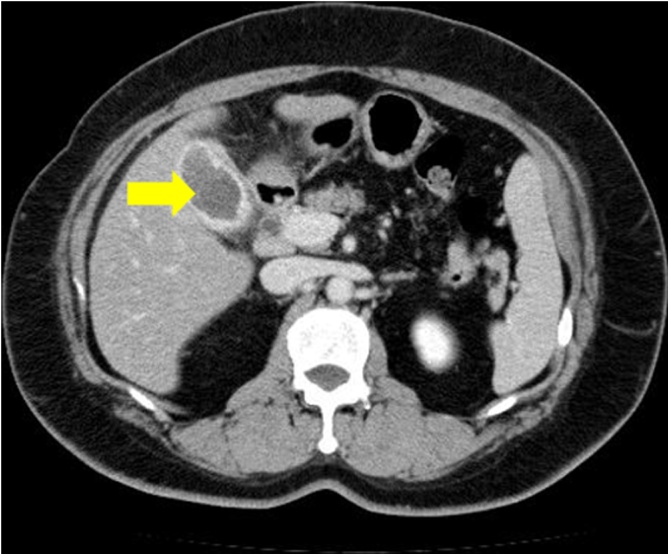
Abdominal computed tomography scan showed a large gallbladder stone (arrow) as a low-density area but no stones in the common bile duct.
